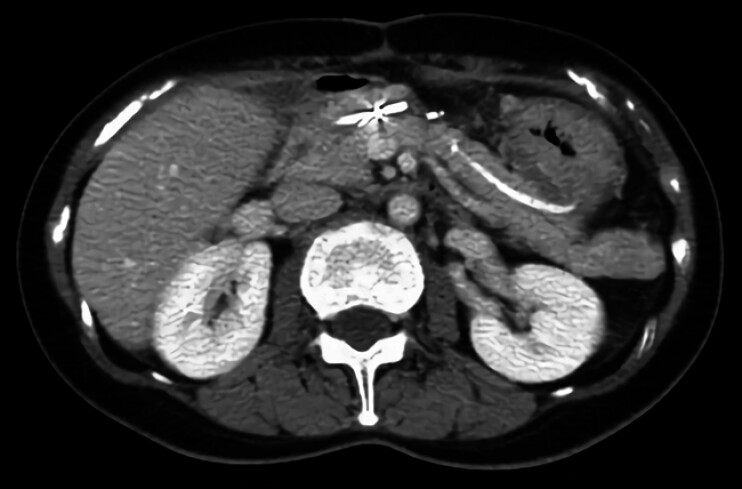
Absence of fluid collection and fistulous tract as seen on the computed tomography scan 6 months after the initial procedure.
